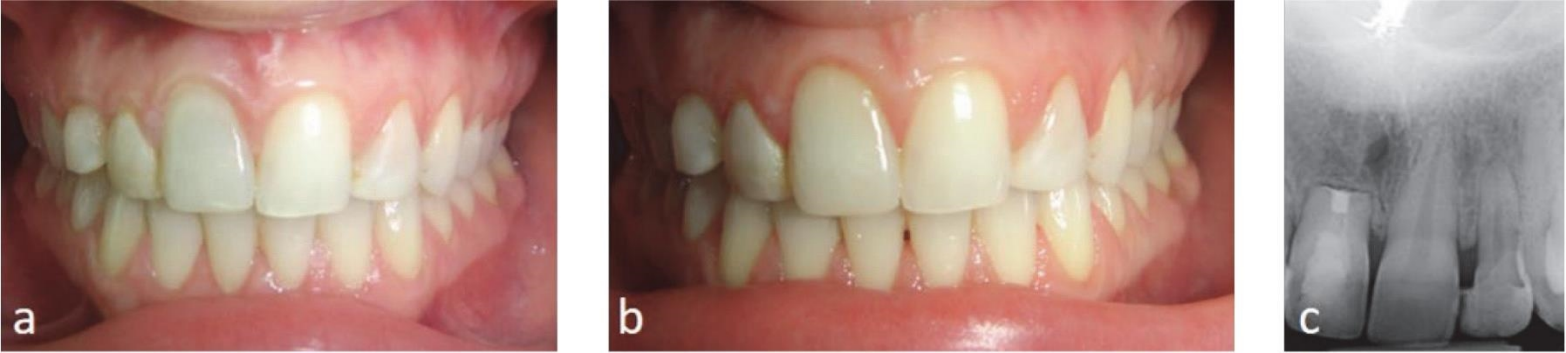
Follow‐up photographs and radiographs 6 years after endodontic microsurgery: (a) mild discoloration 3 years after initial internal bleaching; (b) after repeat internal bleaching; and (c) periapical radiograph showing stable external root resorption.
